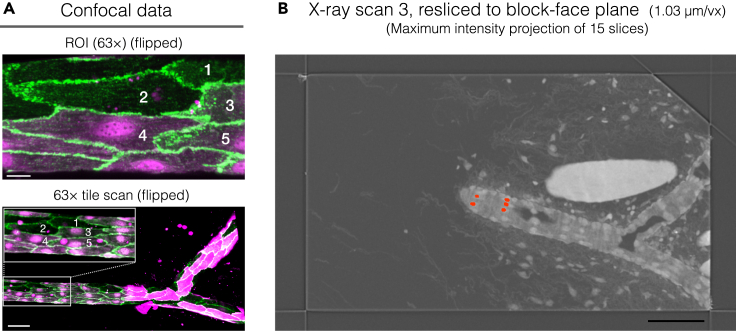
Identification of target cells in X-ray scan 3 Positions of the target cells are annotated with numbers (1–5) in the confocal data (A) of 63× acquisition (top) and 63× tile scan (bottom), and their nuclei are marked red in the X-ray scan 3 (B). Maximum projection of 15 slices (∼15 μm) is shown for better visualization of the target cell nuclei. See also Video S2. Scale bars: (A) 10 μm (top) and 50 μm (bottom); (B) 100 μm.
